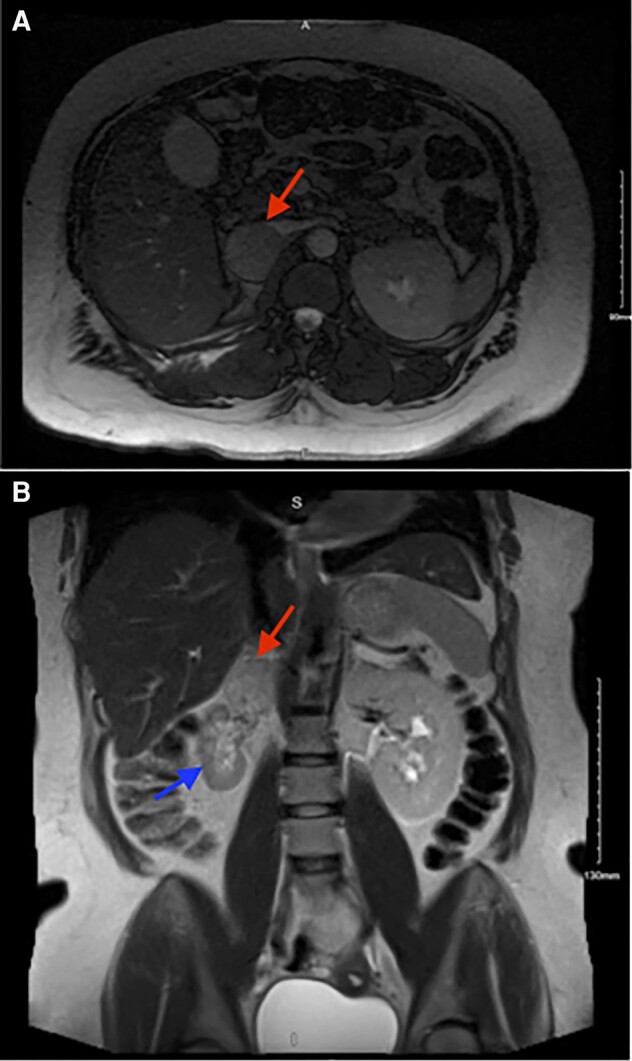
MRI of abdomen and pelvis. (A) Axial section showing 4-cm right adrenal lesion (red arrow) abutting the IVC; (B) Coronal section showing the right adrenal lesion (red arrow) superior to the atrophic right kidney (blue arrow).
